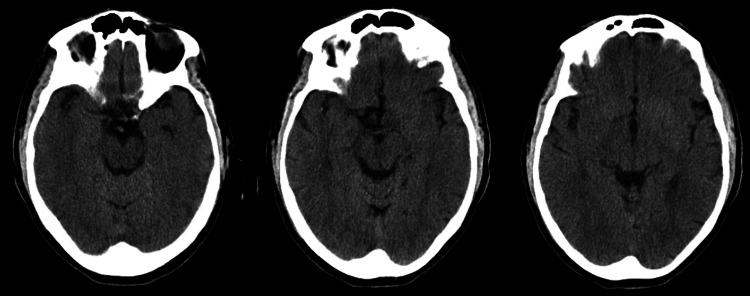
Axial non-contrast enhanced head CT showing absent hyperintensity in the temporal lobes CT: computed tomography
